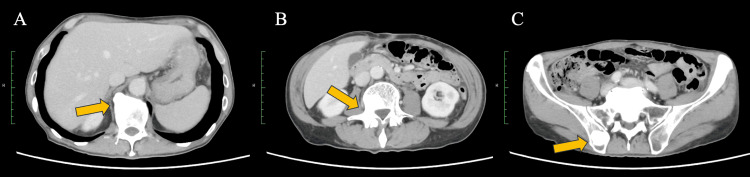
Baseline CT at presentation (A, B, C): The CT shows multiple sclerotic bone lesions involving the vertebral bodies and pelvic bones (arrows).
